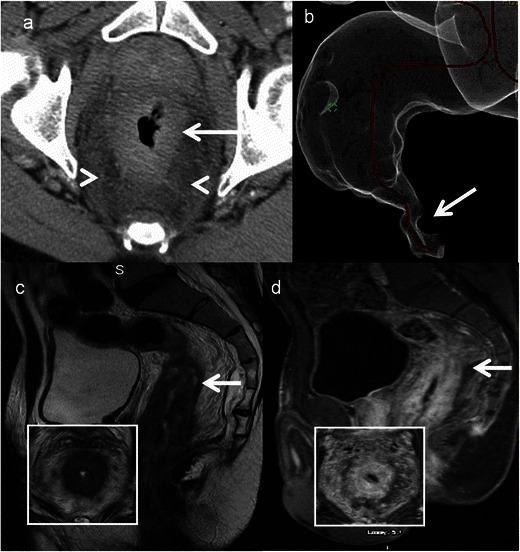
Lymphogranuloma venerum in a 26-year-old HIV-positive man. a Axial CT colonography demonstrates diffuse circumferential thickening of the rectum (*arrow*) with inflammatory stranding in the mesorectum (*arrowhead*). b Reformatted virtual colonoscopy image demonstrates a smooth stricture (*arrow*) with maintained mucosa. c Sagittal T2-weighted MRI and d Sagittal contrast-enhanced T1-weighted MRI reveal circumferential thickening (*arrow*) with luminal compromise of the rectum which is hypointense on T2-weighted MRI and shows enhancement post gadolinium administration. Images in *inset* are the corresponding axial MR images showing similar findings
